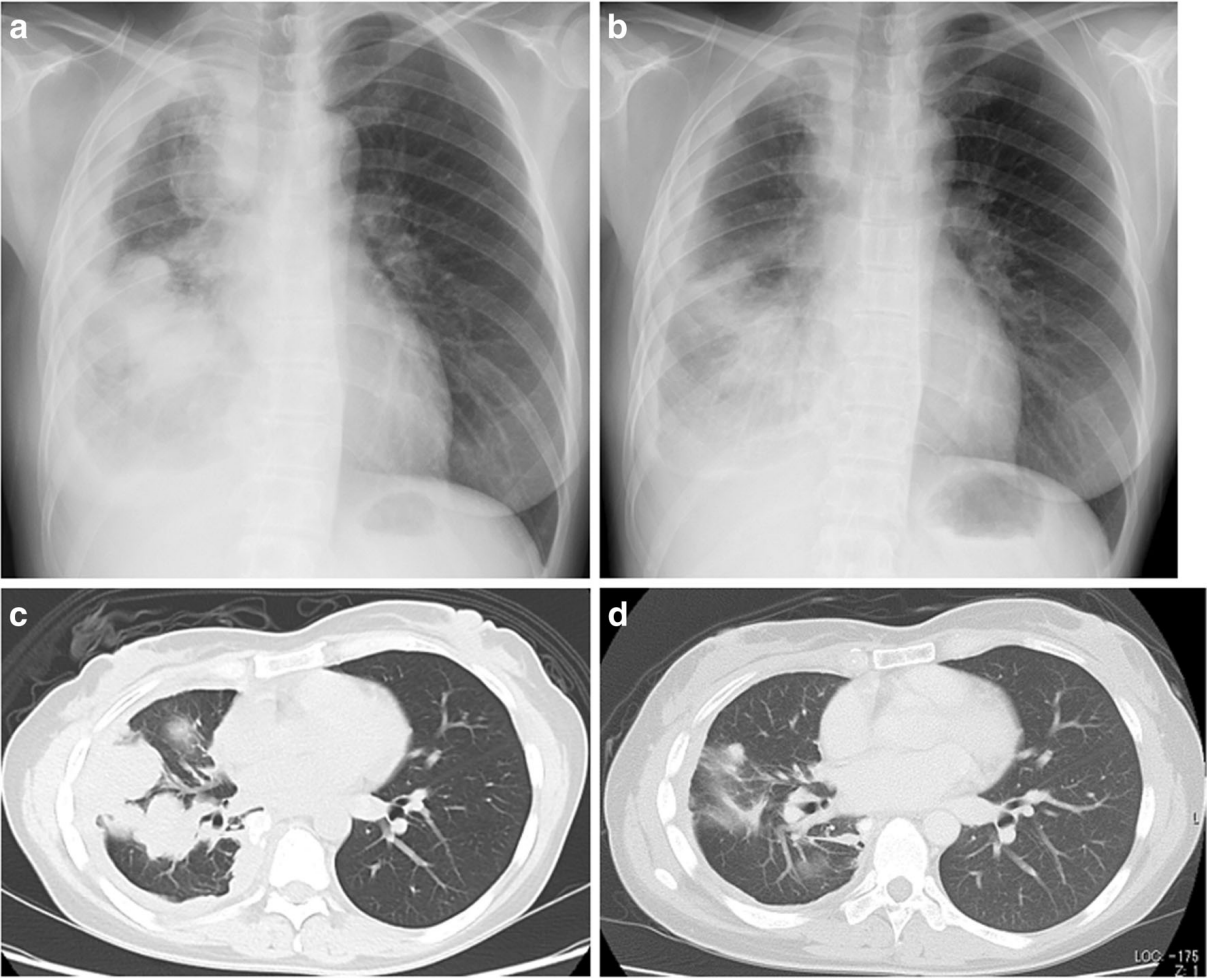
Chest radiographs before and after treatment. a Solid tumors with intrapulmonary metastasis have aggressive regrowth. b Remarkable shrinkage of tumors after osimertinib rechallenge treatment within 1 week. c Solid tumors with intrapulmonary metastasis and no evidence of preexisting interstitial pneumonia before osimertinib rechallenge treatment. d Remarkable shrinkage of tumors after osimertinib rechallenge treatment without evidence of the recurrence of osimertinib-induced interstitial lung disease
